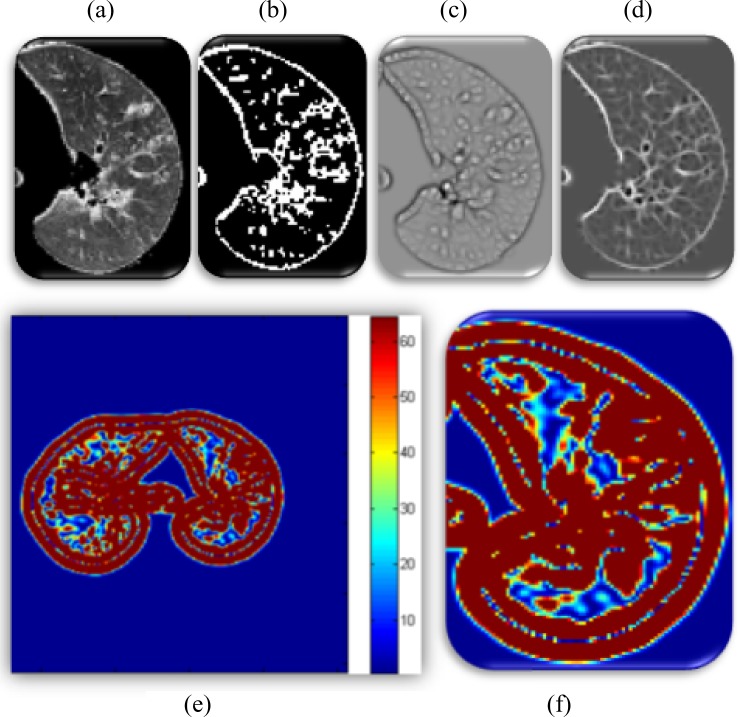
(a) Single-axial CT slice of the left lung. (b) Selected b-scale patterns. (c) Mean Curvature map (}{}$H$). (d) Gaussian curvature (}{}$K$). (e) Willmore energy map. (f) zoomed (e).
